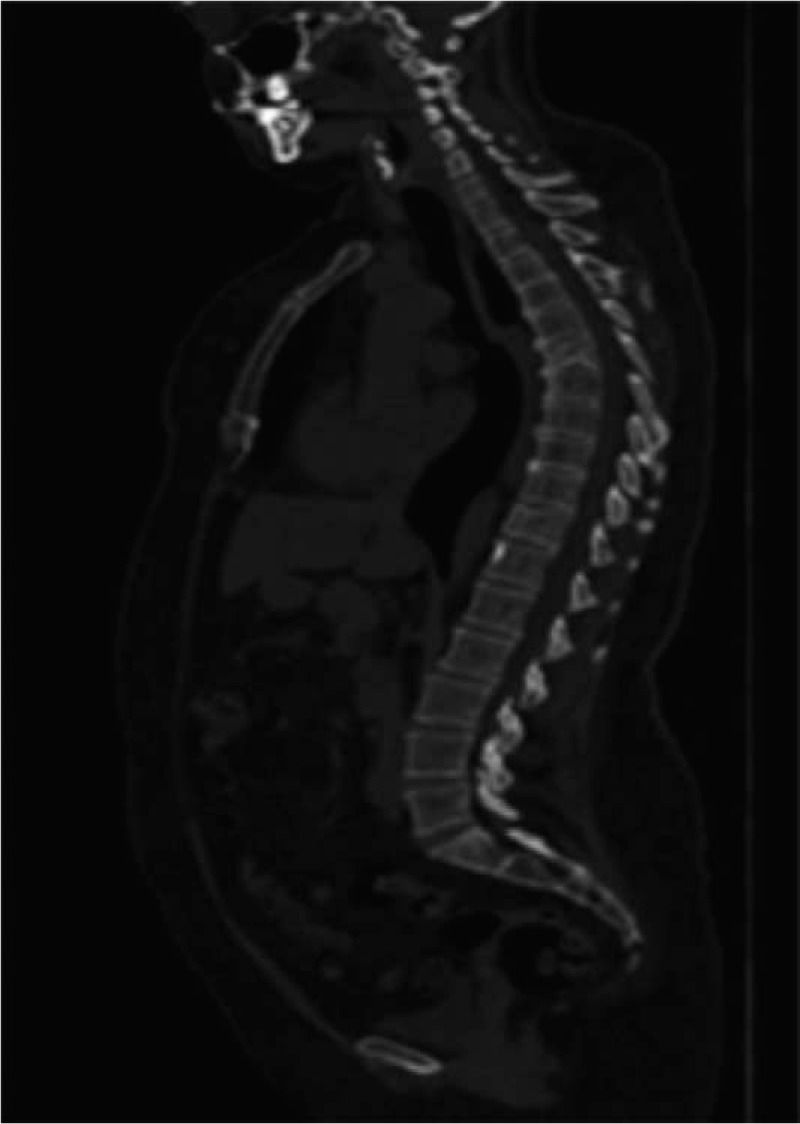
CT scan (bone window) after 12 yr of hormonal treatment for advanced breast cancer (May 2018). CT = computed tomography.
